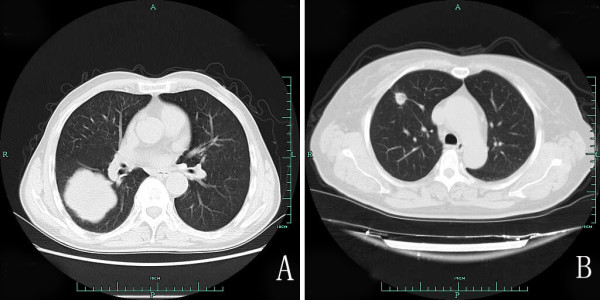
CT scan shows a mass of 8.0 cm in the lower pole (A) and a mass of 1.5 cm in the upper pole of the right lung (B).
